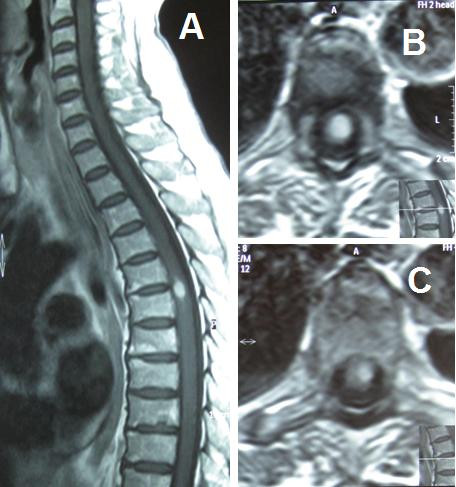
Preoperative MRI: sagittal (a) and axial (b/c) T1 weighted image with contrast showing the intramedullary gadolinium-enhancing lesion at level T5-T6.
